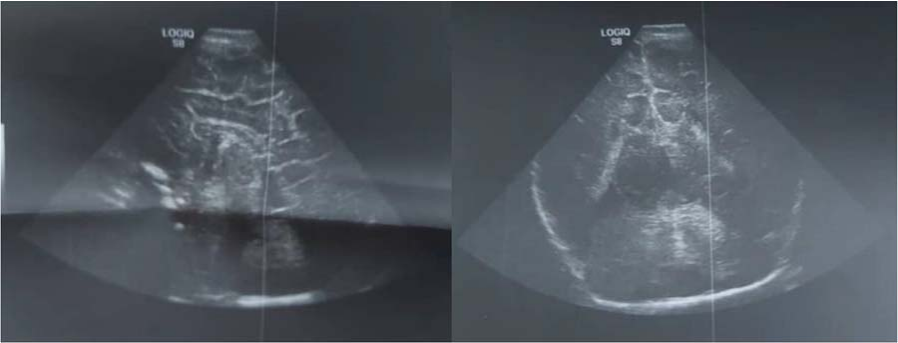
Ultrasound of head shows agenesis of corpus callosum.
